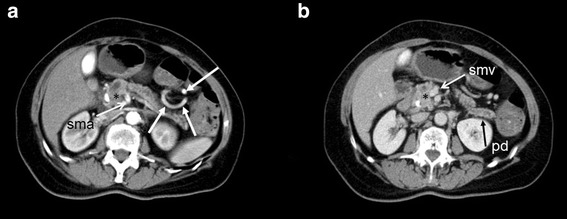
Transverse CT images preoperatively with arterial (a) and venous (b) phases: the pancreatic duct (pd) is dilated, a stent had been implanted into the bile duct due to biliary obstruction of the tumor in the pancreatic head (*). The arterial collaterals are visible in the left upper abdomen (arrows) (sma): superior mesenteric artery, smv: superior mesenteric vein)
